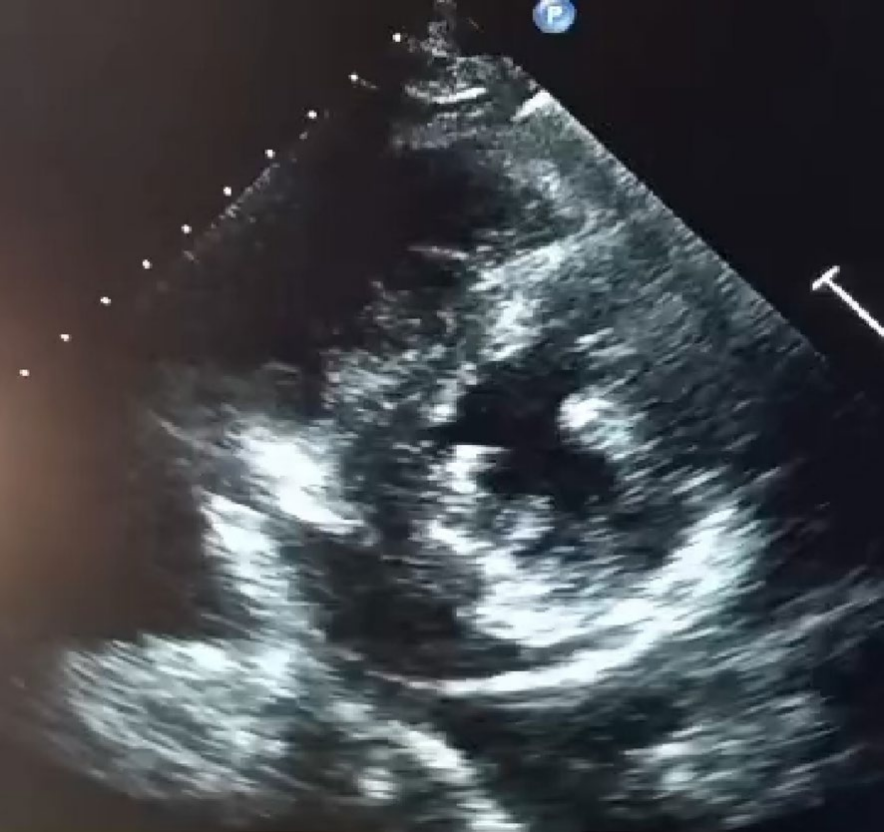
Parasternal short-axis view confirming concentric LV thickening
